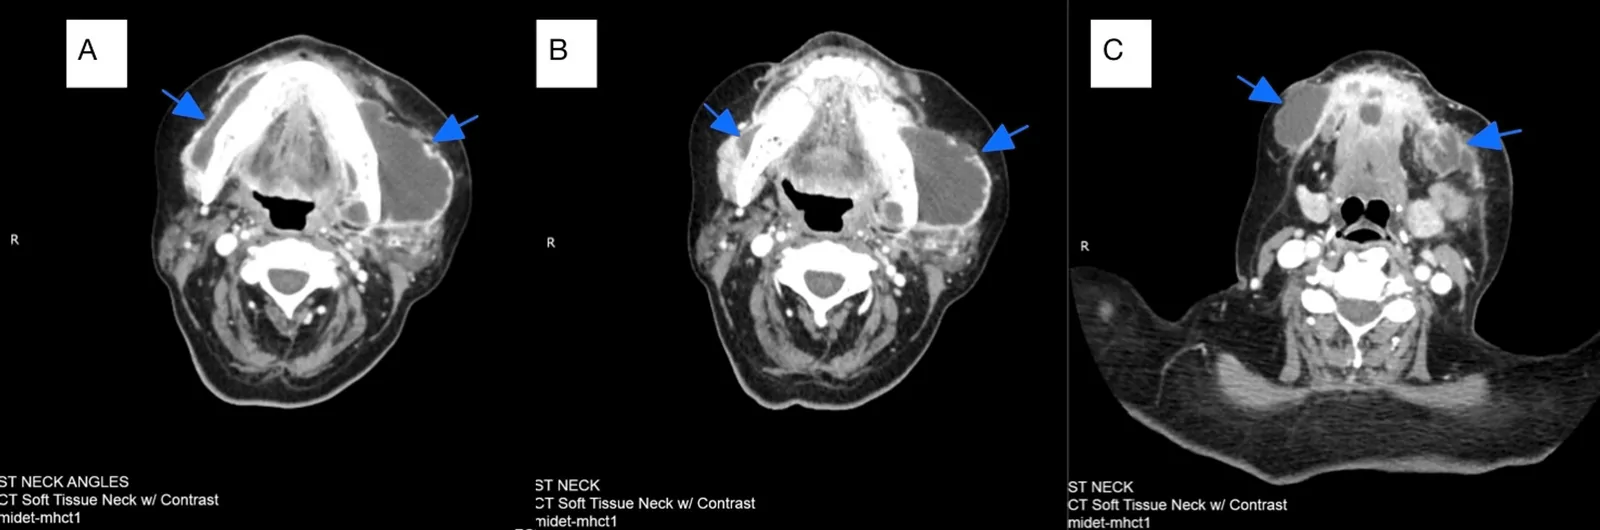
Axial contrast CT of the soft tissue neck and maxillofacial shows bilateral submandibular abscesses formation with suspected mandibular osteomyelitis. Panels A, B, and C demonstrate large complex bilateral submandibular fluid collections (blue arrows).
